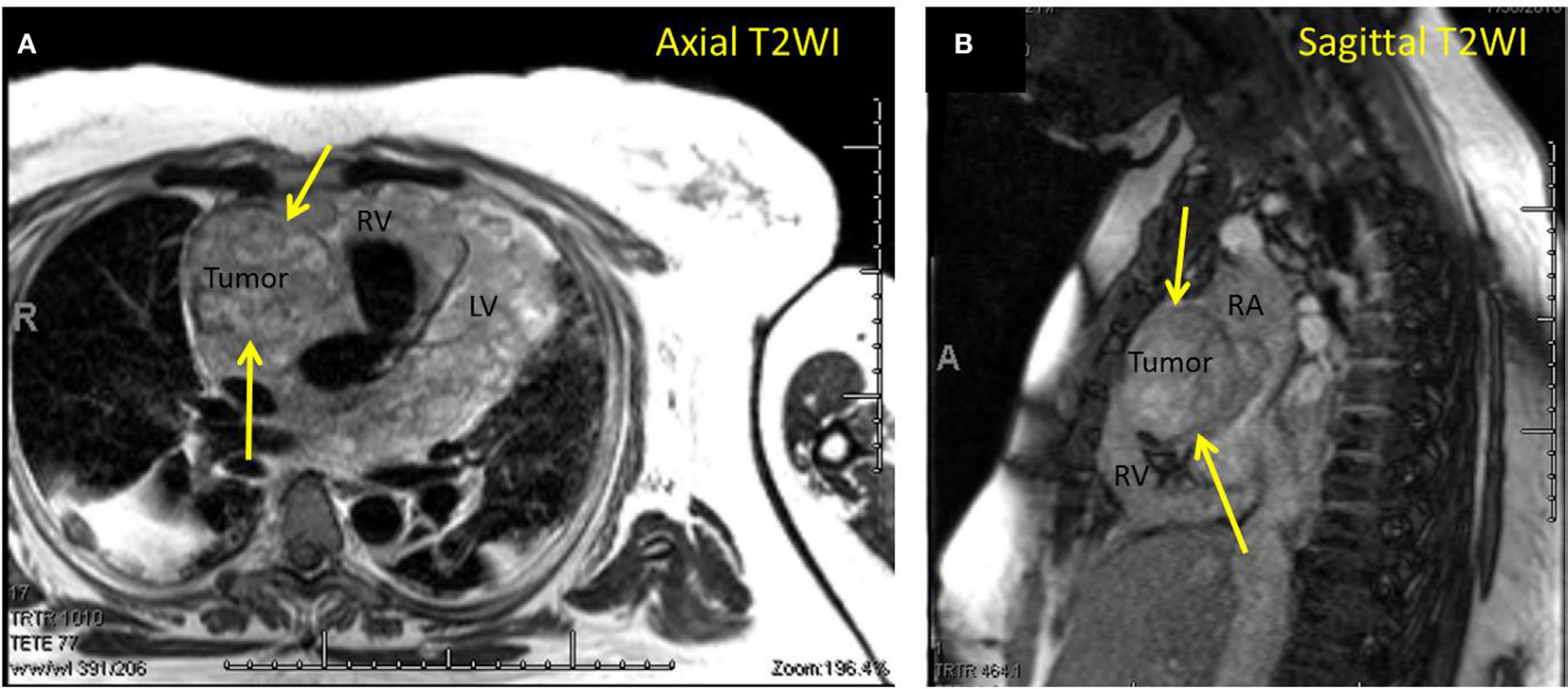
(A) Magnetic resonance imaging (MRI) axial T2-weighted image demonstrating cardiac angiosarcoma. Yellow arrows pointing to tumor. RV, right ventricle; LV, left ventricle. (B) MRI sagittal T2-weighted image demonstrating cardiac angiosarcoma. Yellow arrows pointing to tumor. RA, right atrium; RV, right ventricle.
